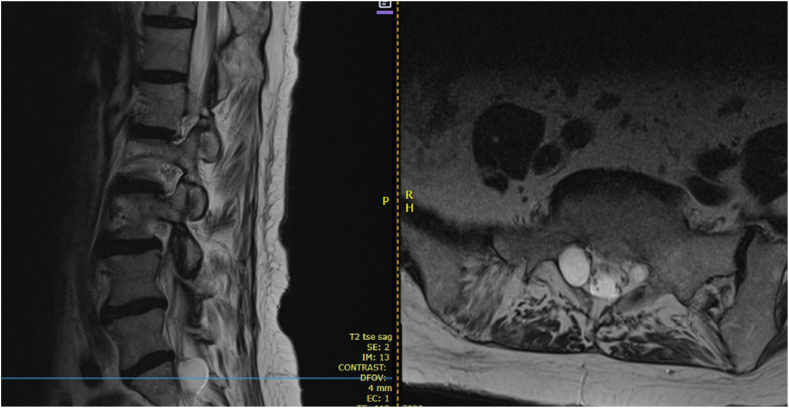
Sagittal and axial views of lumbosacral MRI demonstrating the inferior portion of a Tarlov cyst at S1.
